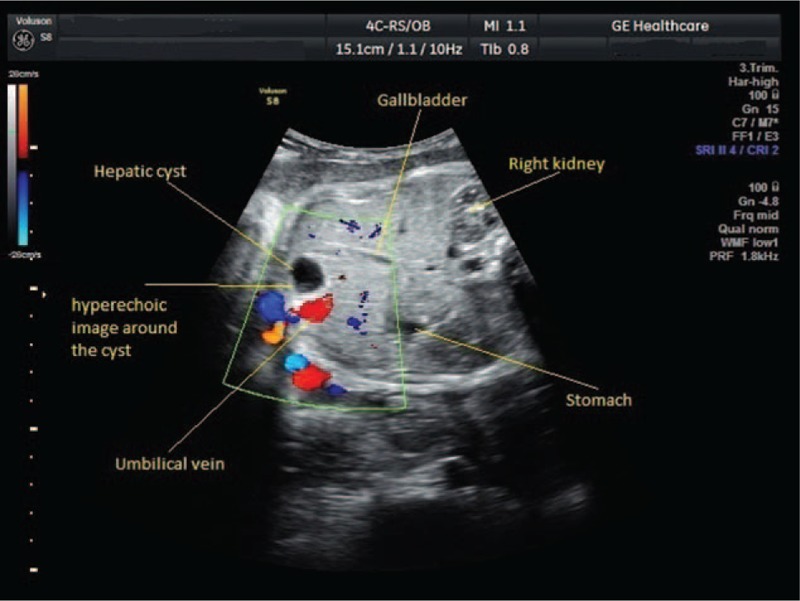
Transabdominal color Doppler ultrasound of a 36-week-old fetus, showing hepatic cyst.
